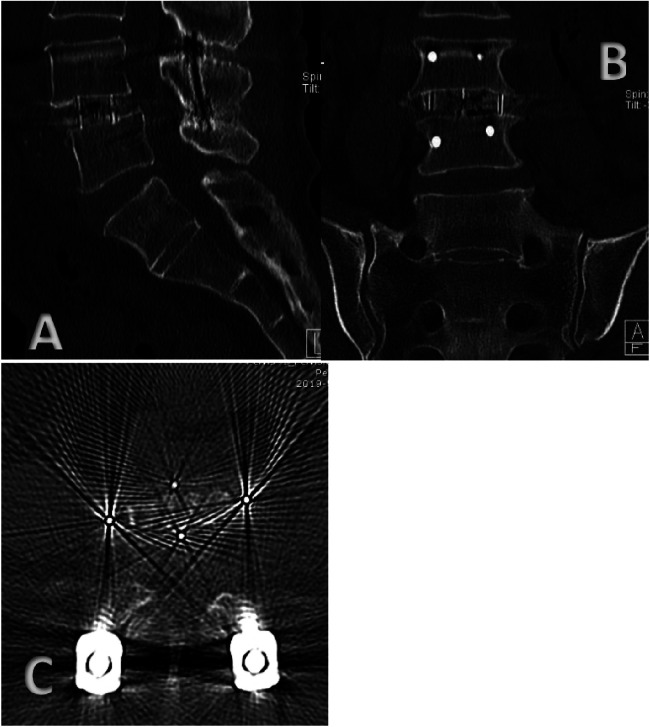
(A) Sagittal CT imaging three years after OLIF combined with percutaneous pedicle screw fixation (PPSF). (B) Coronal CT imaging three years after OLIF combined with percutaneous pedicle screw fixation (PPSF). (C) Transverse CT imaging three years after OLIF combined with percutaneous pedicle screw fixation (PPSF).
